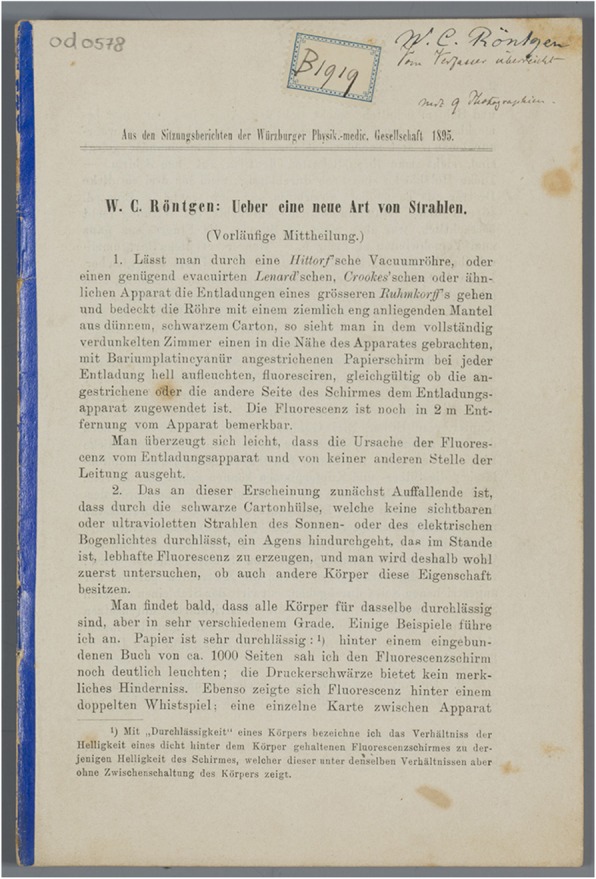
Reprint of the first publication by Roentgen on his discovery of a new kind of rays. This is the reprint with the blue rim on its spine that was sent to Lorentz
